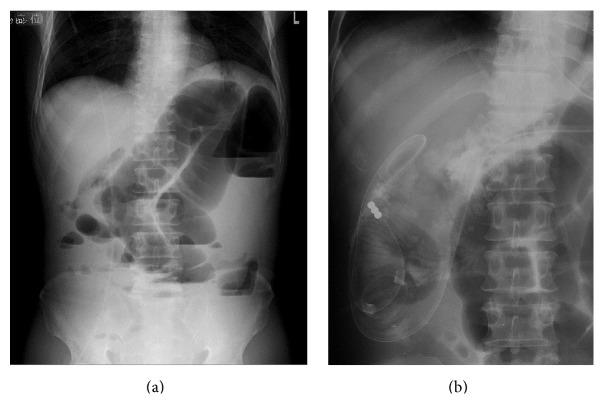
An abdominal X-ray examination revealed the dilation of the colon and niveau (a). At the insertion of an ileus tube, the C-loop of the duodenum was observed to be absent and the small intestine was located on the right side of the abdomen (b).
